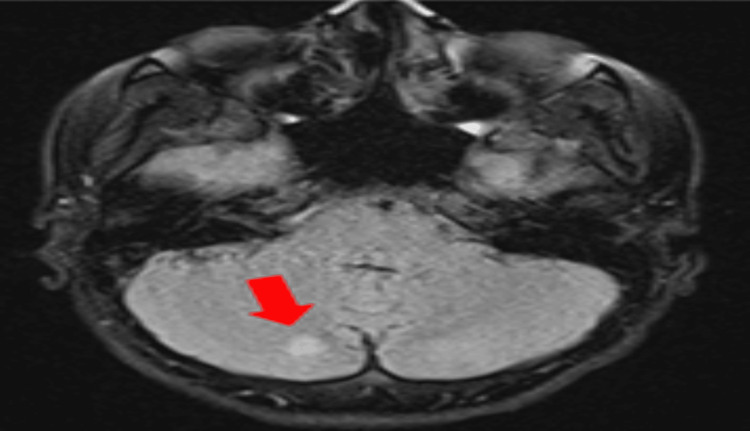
Control cranial MRI A new embolus was identified in the inferior region of the right cerebellar hemisphere MRI: magnetic resonance imaging
